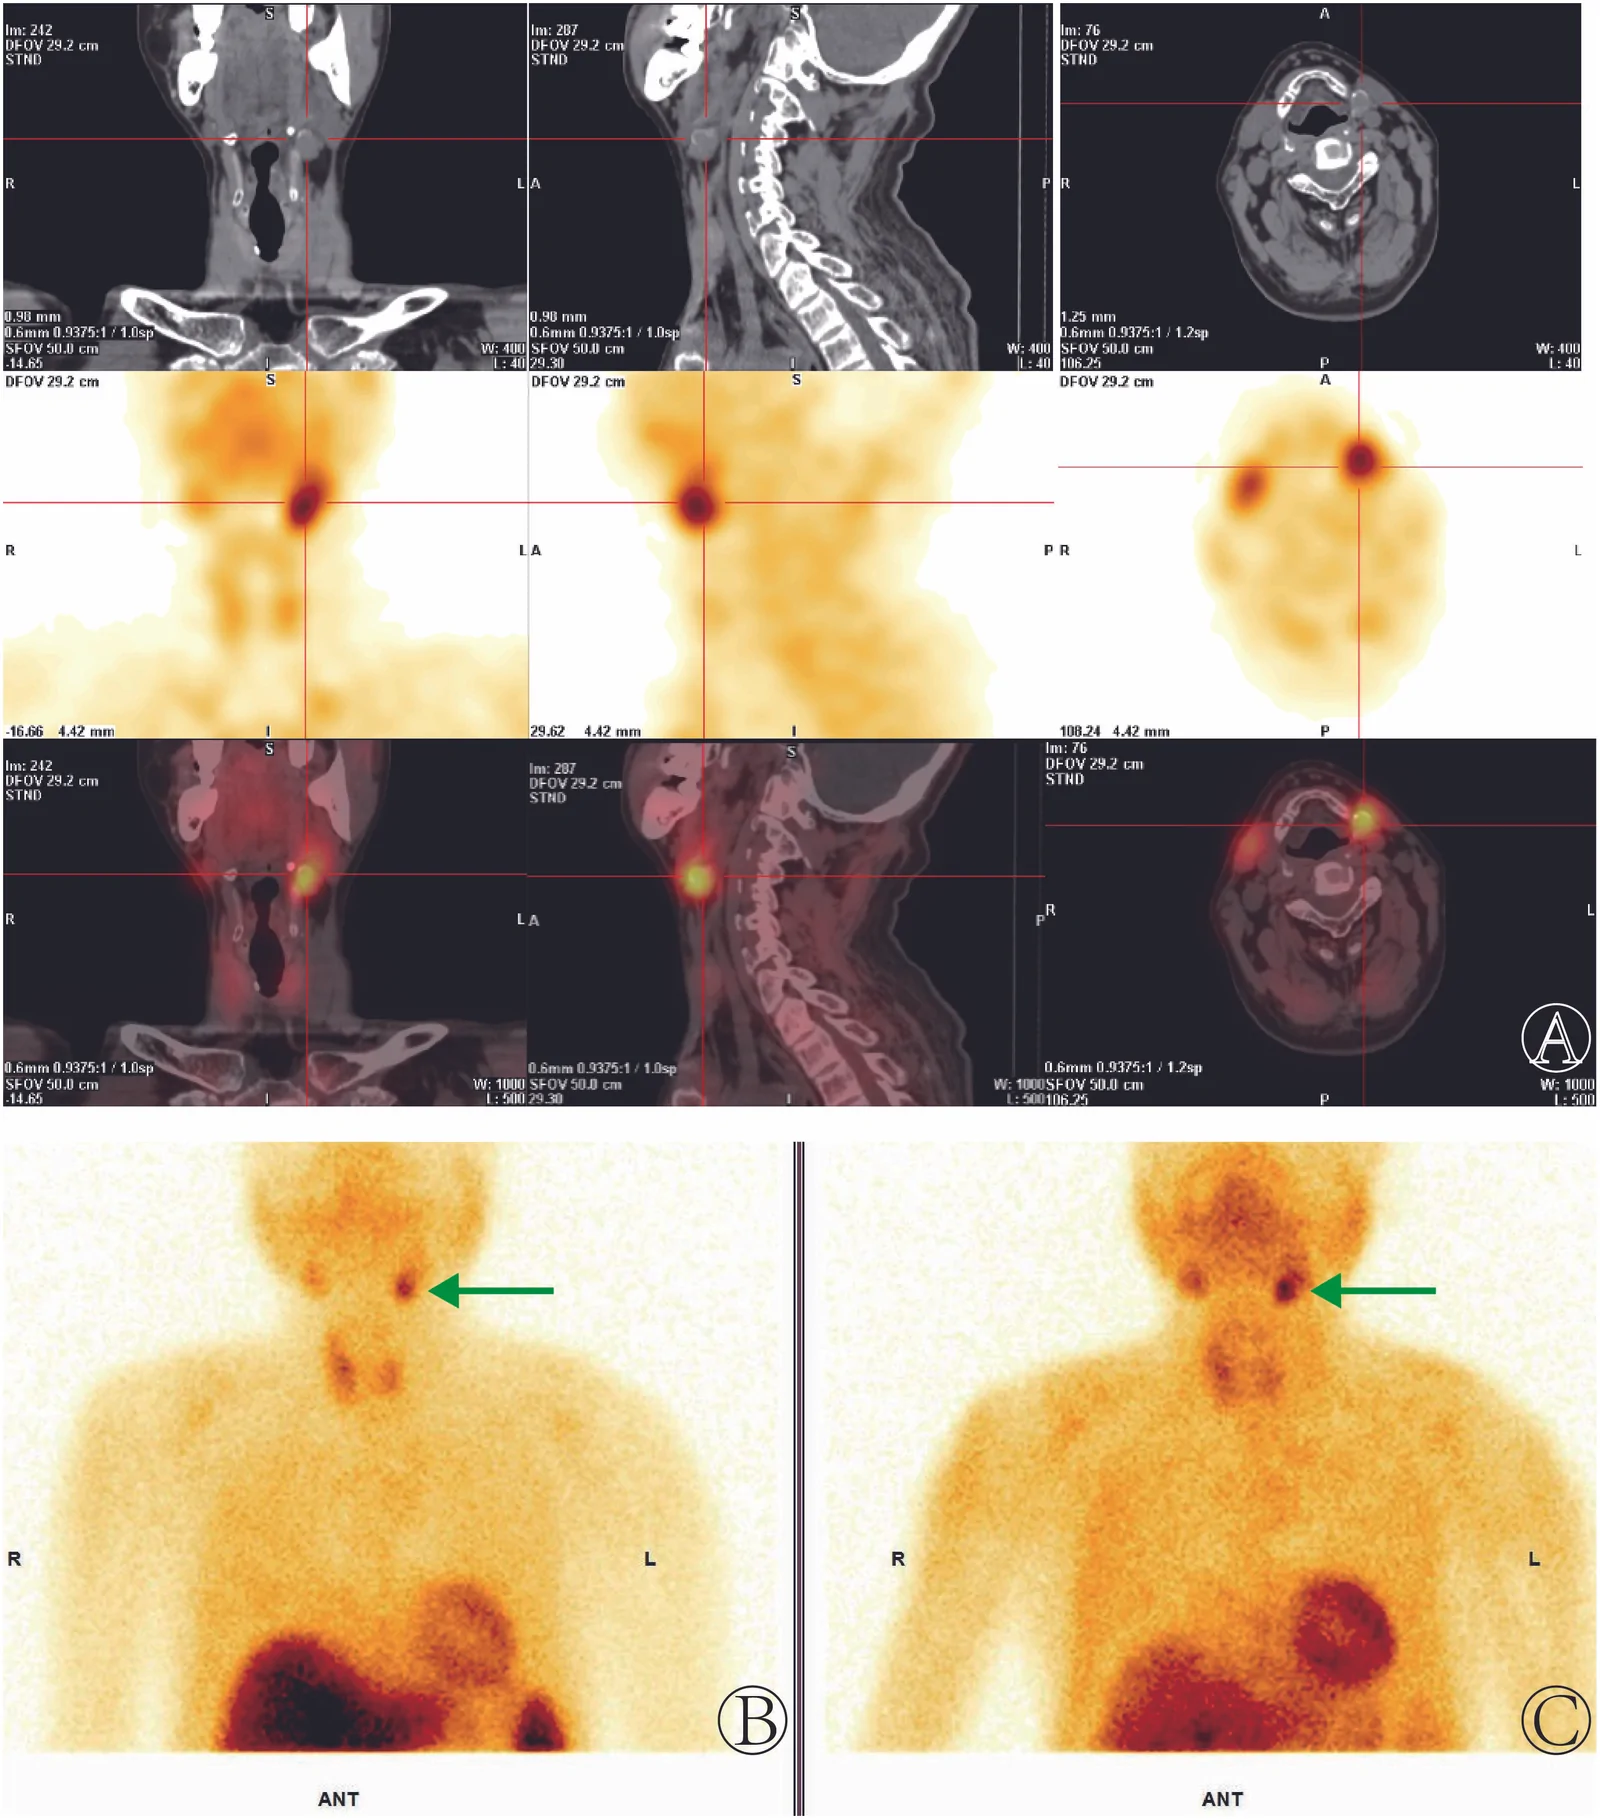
^99m^Tc-MIBI SPECT/CT fusion images and early and late planar images of ^99m^Tc-MIBI SPECT for case 1. (A) First row: Three-dimensional CT scan images; second row: ^99m^Tc-MIBI SPECT images; third row: ^99m^Tc-MIBI SPECT/CT fusion images. The first, second, and third columns show the coronal, sagittal, and transverse planes, respectively. (B) Early (20 min) planar image of ^99m^Tc-MIBI SPECT: Increased tracer uptake (arrow) in a nodule in the medial left submandibular region (level IIa). The thyroid gland is visible inferiorly. (C) Late (2 h) planar image of ^99m^Tc-MIBI SPECT: Persistent increased tracer uptake (arrow) in the nodule in the medial left submandibular region (level IIa). Tracer uptake in the thyroid gland is generally decreased compared with the early image.
